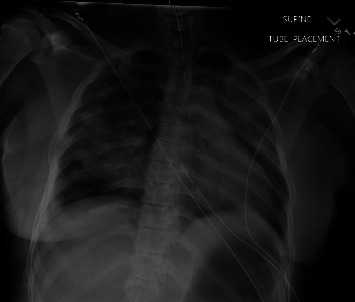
Postintubation chest X-ray showing worsening infiltrates bilaterally.
